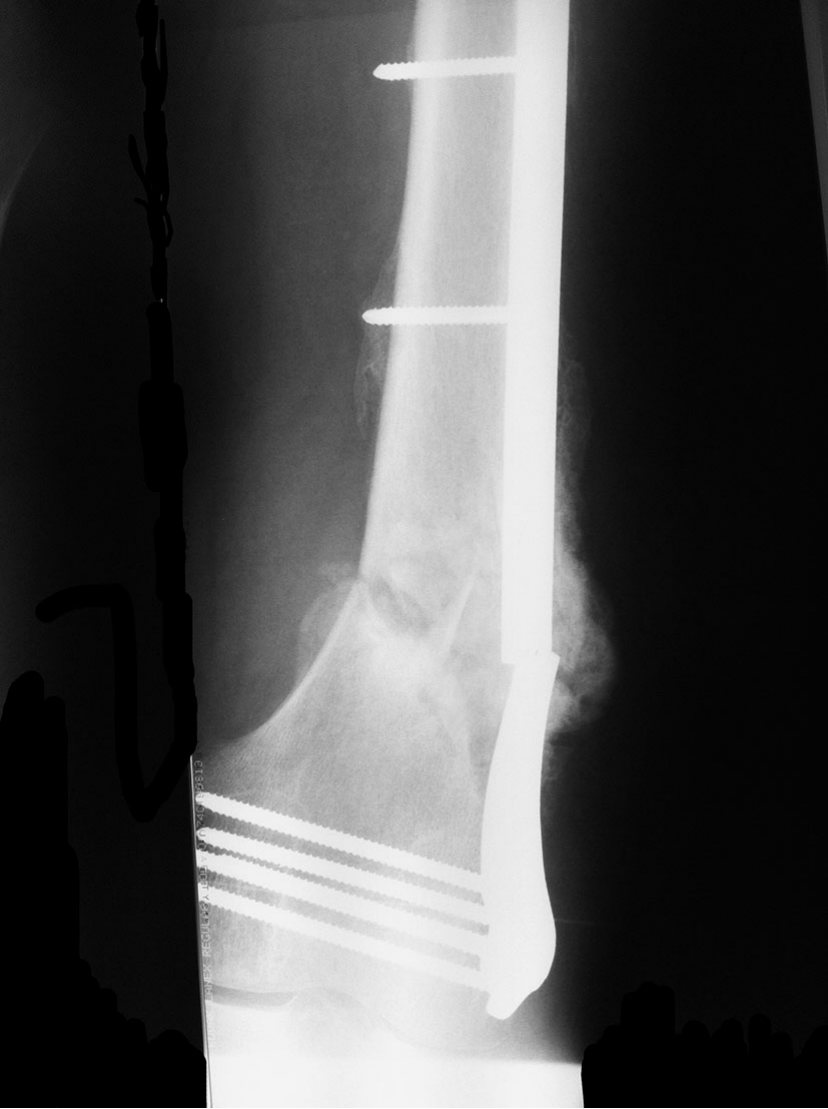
Anteroposterior radiograph of the distal femur demonstrating angulation, nonunion and failed locking plate at the fracture site
